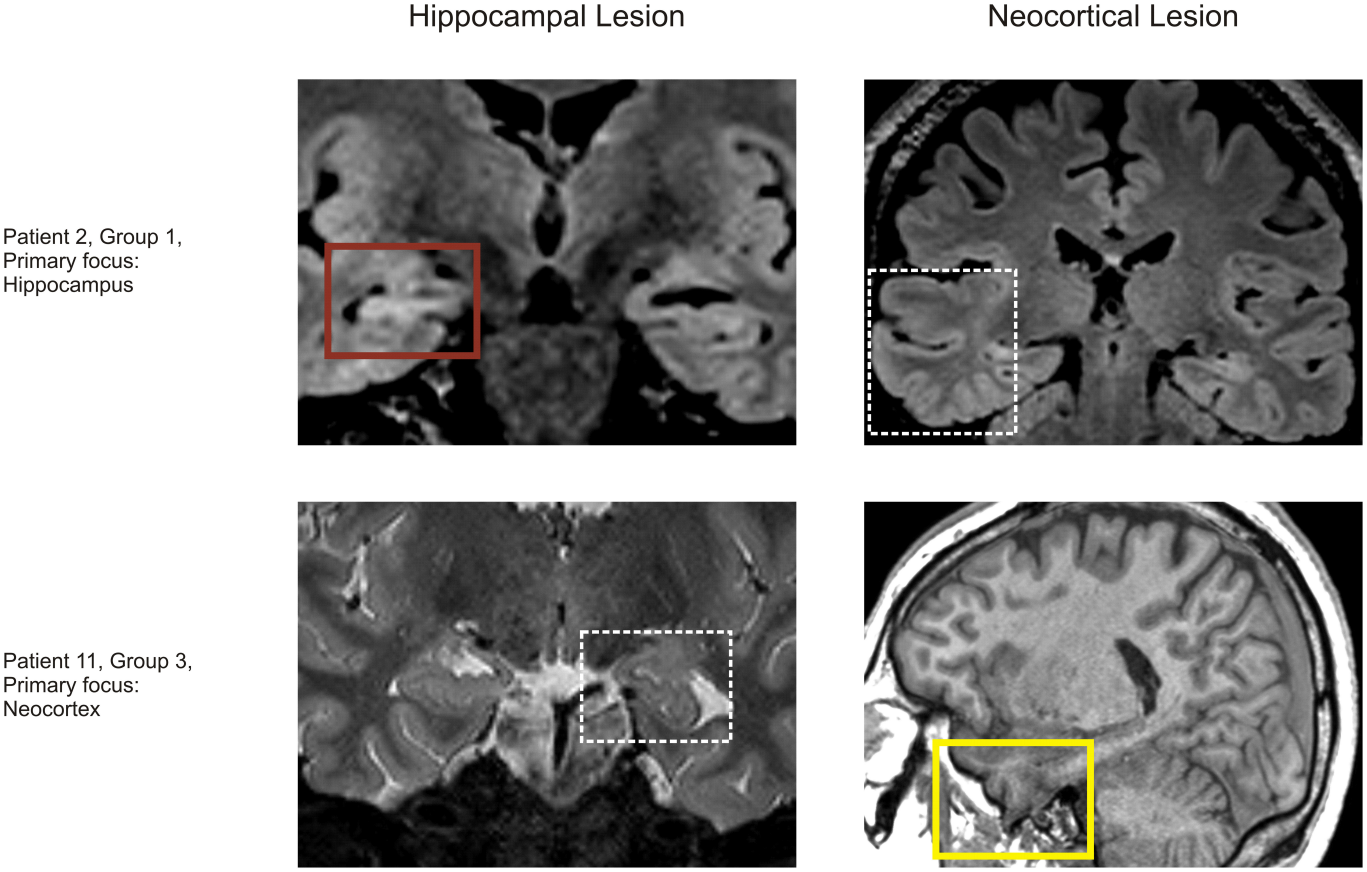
Two exemplary patients. (Upper row) Patient 2 had her primary focus in the hippocampal lesion. MRI showed hippocampal sclerosis (upper left) and a temporal lobe FCD (upper right, white box). Ictal SEEG suggested that seizures were only generated in the hippocampal lesion and interictal fast ripples were more frequent in the hippocampus (R = 0.61; red box). (Lower row) Patient 11 had his primary focus in the neocortical lesion. MRI showed a hippocampal malrotation (lower left, white box) and a temporal lobe meningoencephalocele (lower right). Ictal SEEG suggested that seizures were only generated in the neocortical lesion and interictal fast ripple rate was more or less similar (in this case slightly higher in the neocortical lesion; R = −0.05; yellow box).
